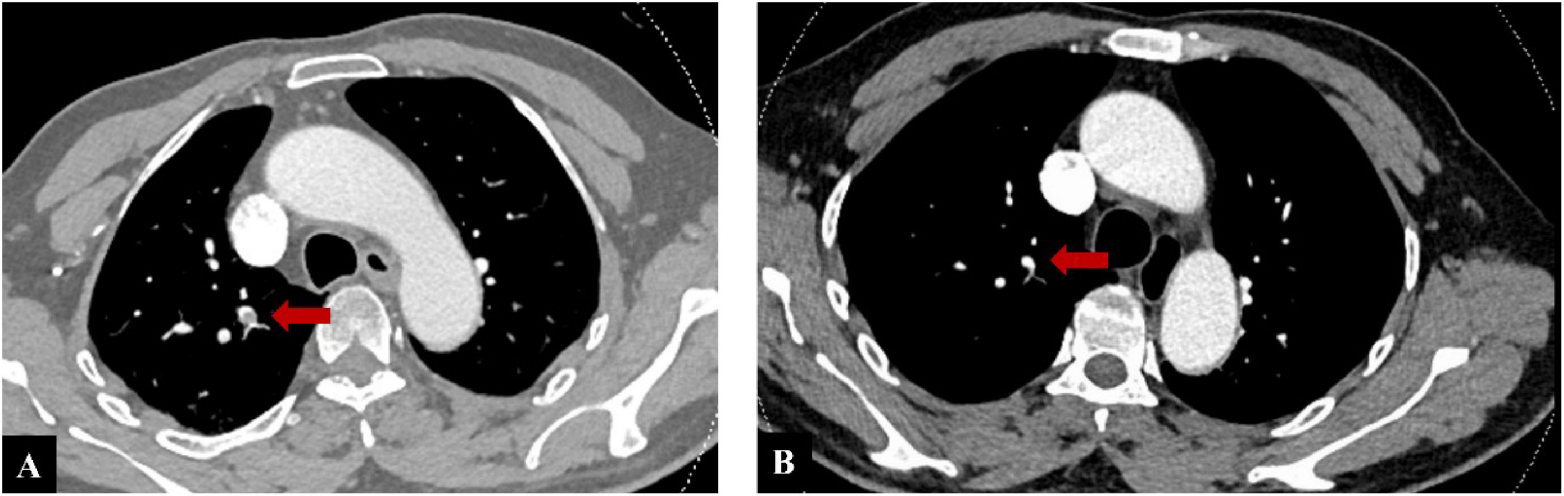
(A) Computed tomography pulmonary angiography (CTPA) on admission, red arrow demonstrating thrombus formation in the right pulmonary artery. (B) Follow-up CTPA at the 6-month evaluation, demonstrating complete disappearance of the right pulmonary artery thrombus.
